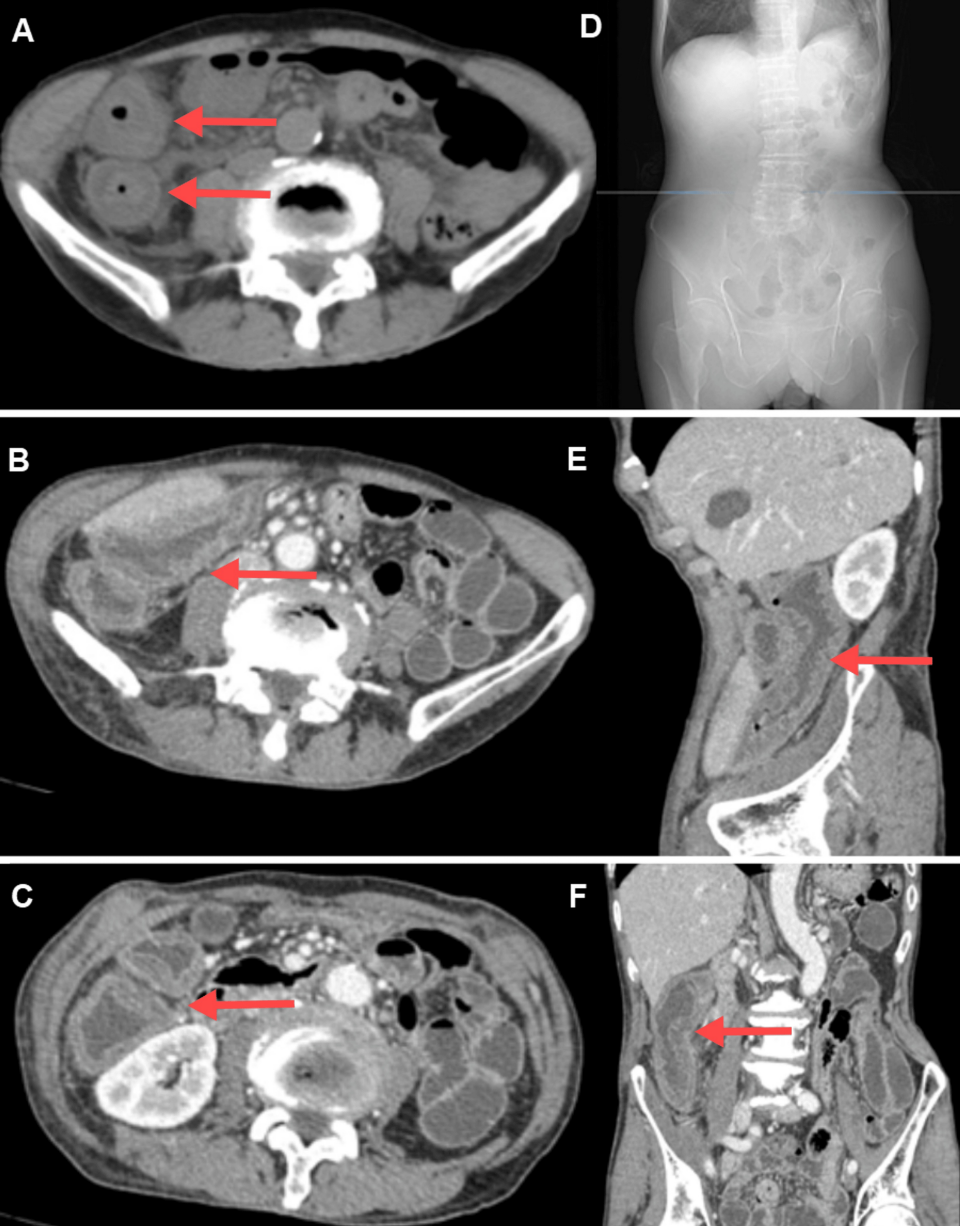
CT Imaging of Colonic Wall Thickening and Edema in the Ascending Colon and Ileocecal Region (Red arrow point). (A) Non-contrast CT shows significant wall thickening in the ascending colon in the right mid-abdomen, with luminal narrowing and a maximum wall thickness of approximately 13 mm. A faint “double halo” sign is visible (left). (B) Contrast-enhanced CT at the hepatic flexure of the colon (left). (C) Contrast-enhanced CT at the ascending colon (left). (D) The blue line indicates the level of panel A on the X-ray image, which shows no air-fluid levels or signs of obstruction. (E) Sagittal view.
